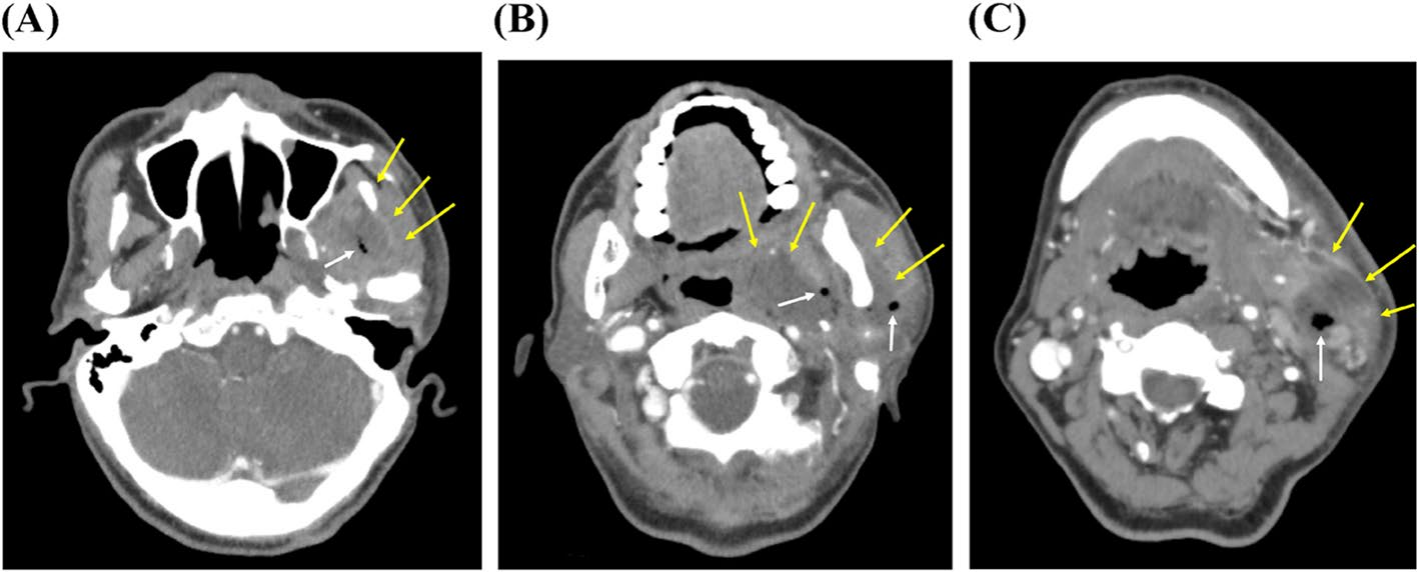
Neck angiography CT images 5 days after the first visit (case 2). Extensive heterogeneous lesions of the fascial space and muscles (yellow arrows) with inner aerobic voids (white arrows) were observed. A Masseteric space. B Parapharyngeal and masseteric space. C Submandibular space
